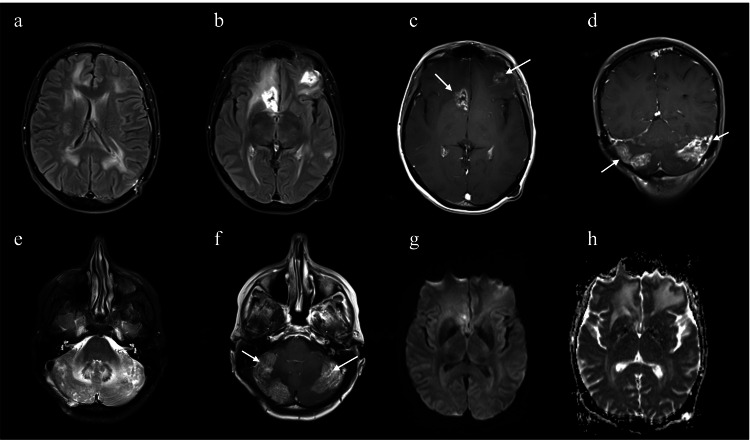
An MRI done two weeks before the stroke onset demonstrated the patient's known supra- and infratentorial metastatic infiltration, with enhancement (white arrows), edema, and mass effect. Diffusion-weighted imaging (DWI) (g, h) did not show any diffusion restriction to suggest an infarct.
